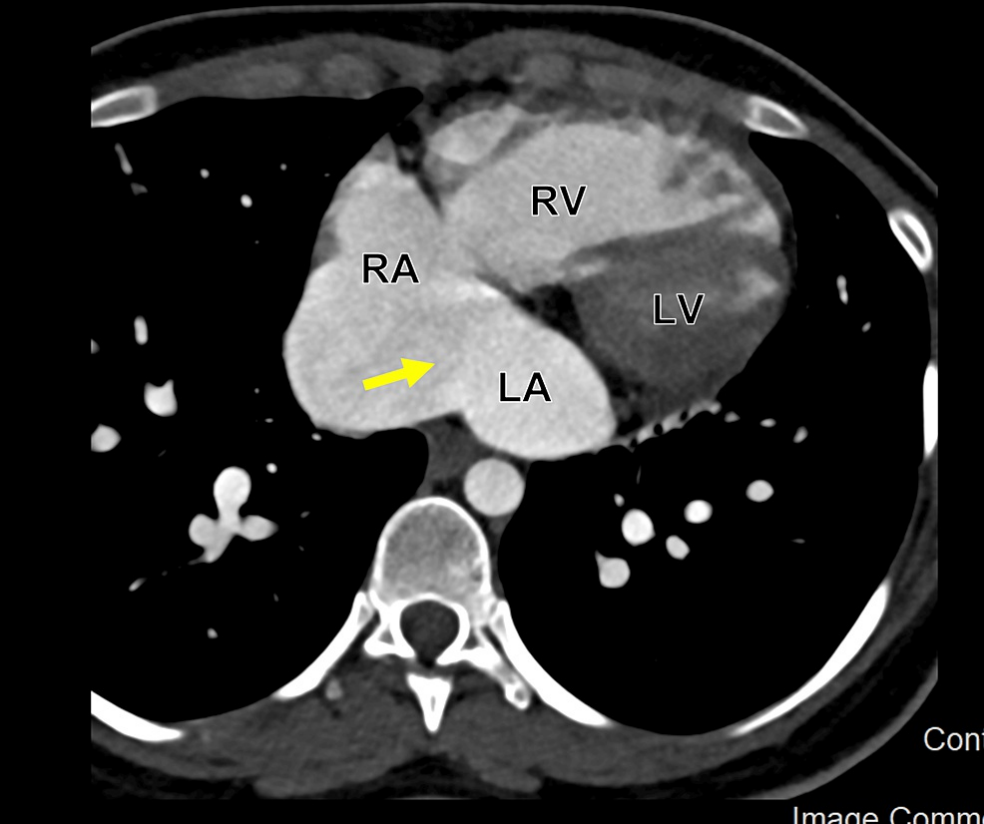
Cardiac computed tomography angiography (CCTA) in axial display showing a communication between the RA and LA representing ASD. RA: Right atrium; LA: Left atrium; ASD: Atrial septal defect.
